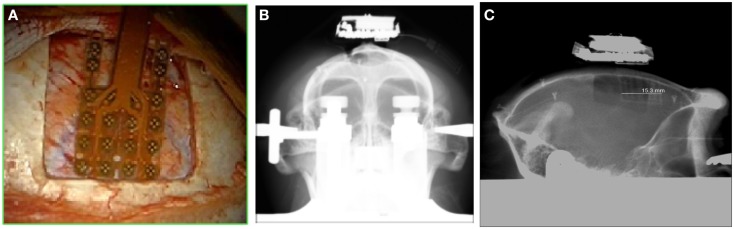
Location of the grid on the cortex (A); frontal X-ray showing the position of the whole device (B) and the lateral view (C).
